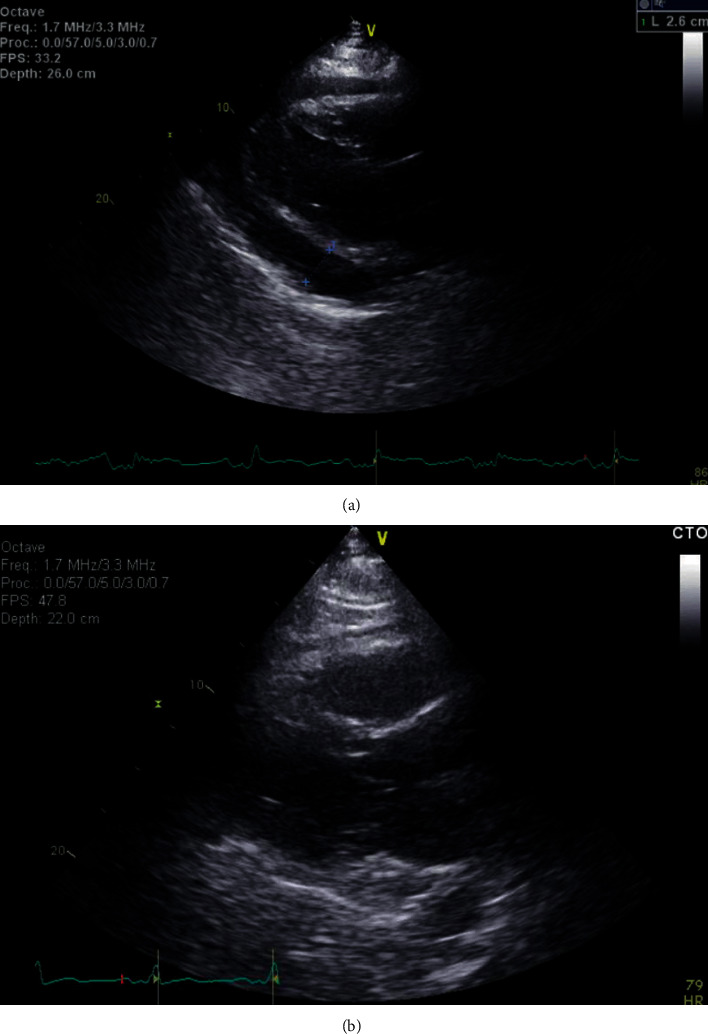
(a) Large circumferential pericardial effusion measuring 2.6 centimeters posteriorly, 1.5 centimeters anteriorly, and 1.8 centimeters at the apex with right atrial collapse and without right ventricular collapse. Significant inflow variation was noted across the mitral and tricuspid valves. Left ventricular ejection fraction was estimated at 55%. Mild concentric left ventricular hypertrophy was present. (b) Echocardiogram obtained the day after pericardial drainage demonstrates resolution of the pericardial effusion.
